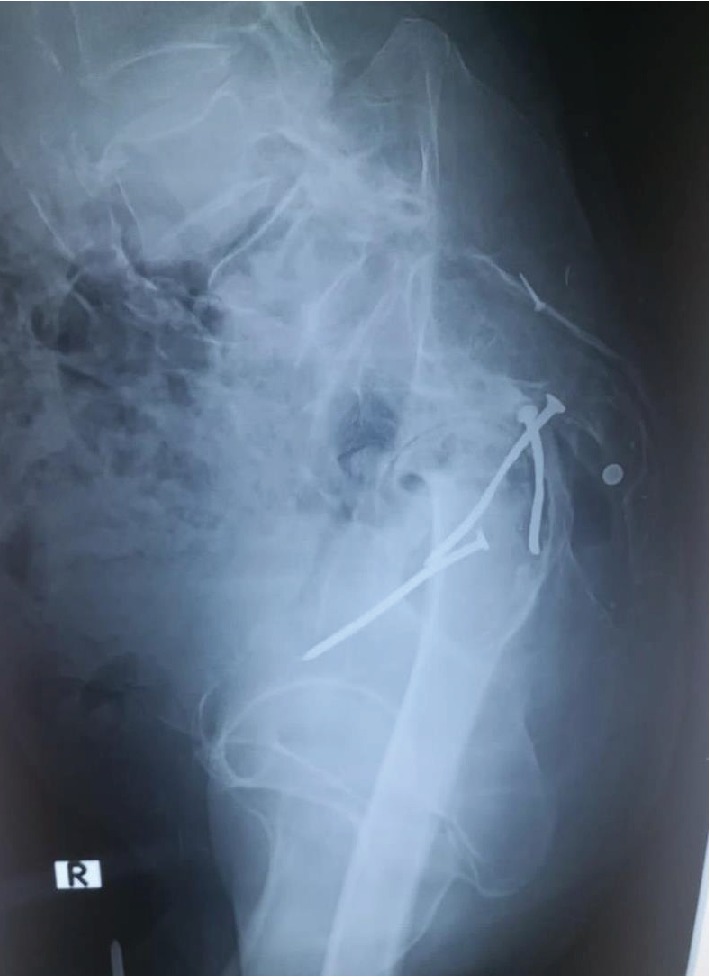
X-rays of pelvis and right hip joint lateral view showing grade 4 osteoarthritis of the right hip joint with destruction of the right femoral head due to retained shrapnel.
